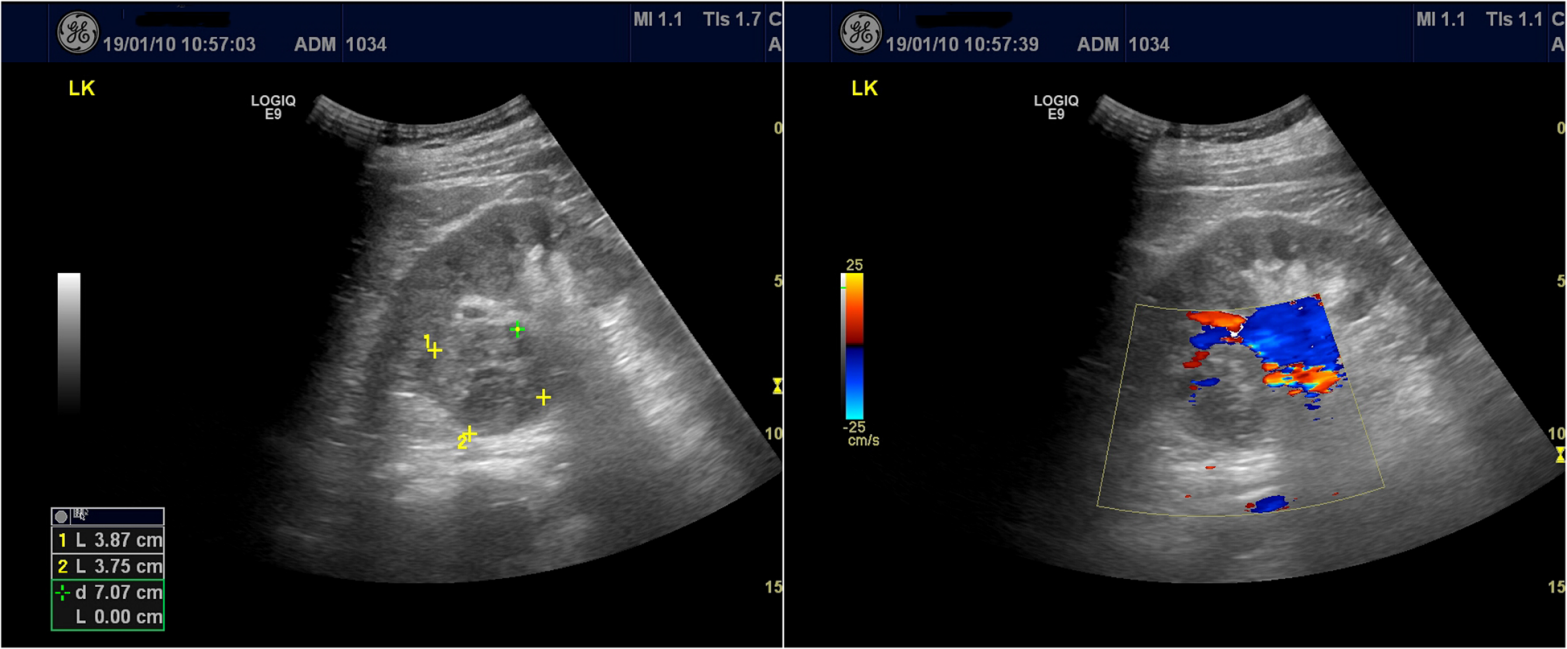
Doppler flow imaging ultrasonography (CDFI) in a 29-year old man shows a regular hypoechoic mass, 3.8×3.7 cm in size, with distinct borders in the upper pole of the left kidney and dot or strip-like flow signal
